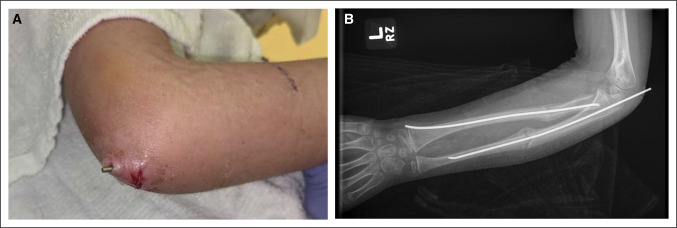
Clinical photograph (A) and anteroposterior radiograph (B) showing the nail position at the 4-week follow-up appointment.
